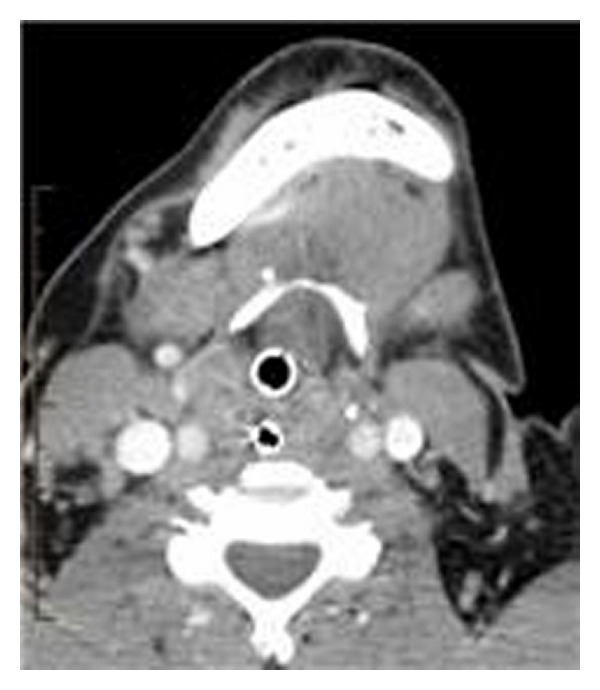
CT scan in the axial plane revealing near-complete airway obstruction at the level of the oropharynx.
